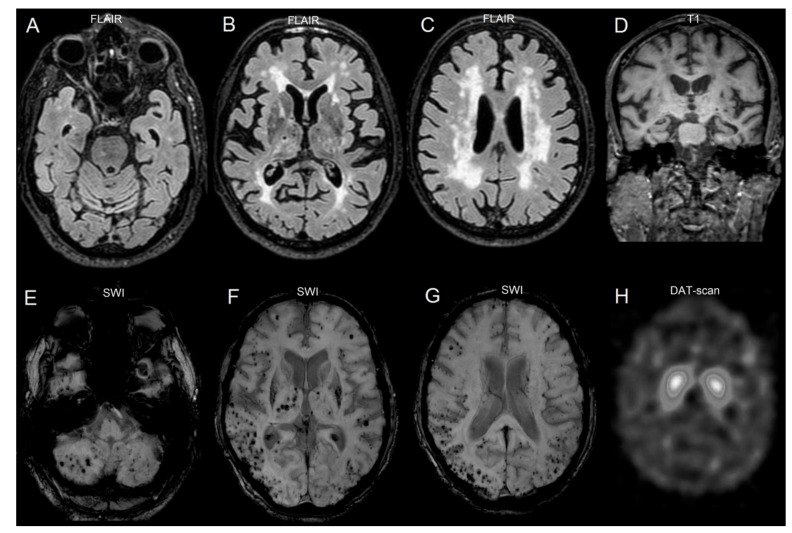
Neuroimaging of the patient. Axial Fluid Attenuation Inversion Recovery (FLAIR) images indicate white matter hyperintensities from the level of the pons up to corona radiate (A–C), while some lacunes are also present at the level of basal ganglia and thalami (B). Despite neocortical atrophy shown in B and C, coronal T1 image (D) indicates relative preservation of the hippocampus, but additional presence of some lacunes. Axial susceptibility weighted images (SWI) show multiple microbleeds in the cerebellum (E), basal ganglia (F) and cortical-subcortical locations with a posterior preference (F,G). DAT-scan (H) indicates reduction of dopamine transporter activity, with some degree of asymmetry.
